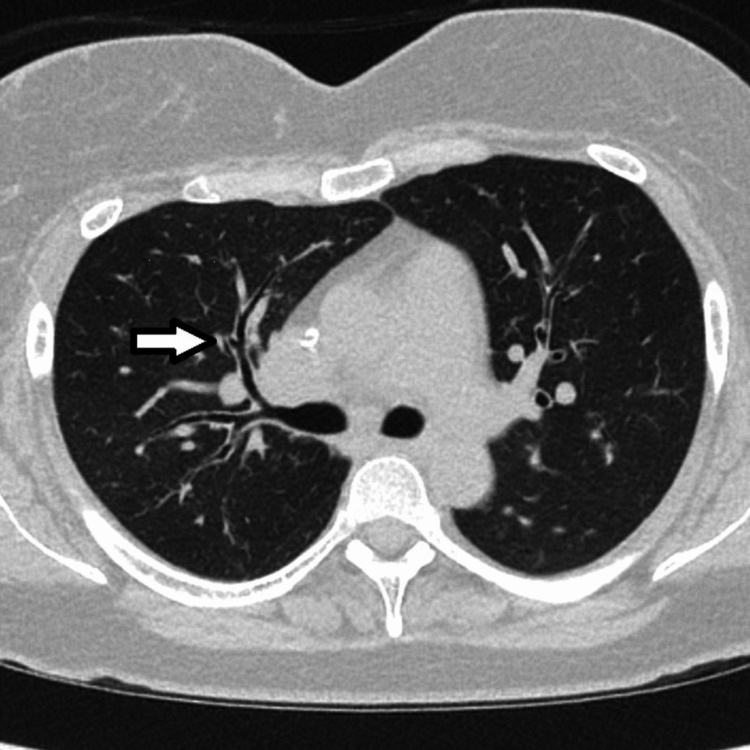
CT of the chest. Lung window. A level below the carina. Right middle lobe bronchiectasis (arrow).
